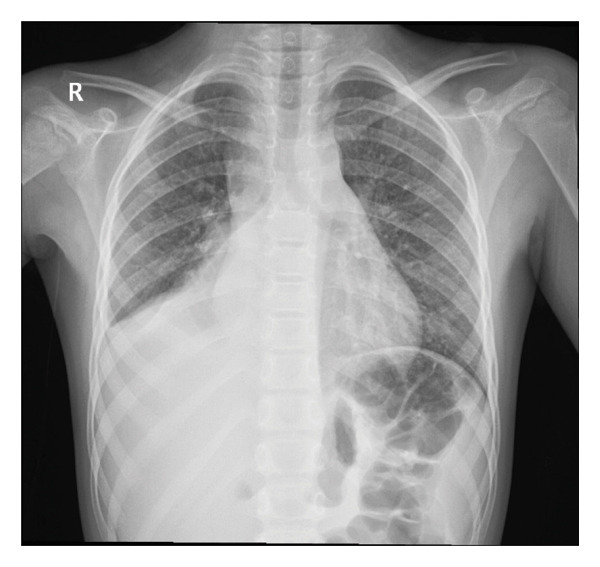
Posteroanterior (PA) chest radiograph shows increased homogeneous opacification of the right hemithorax with a lateralized pleural line and poor delineation of the right hemidiaphragm and right cardiac border, consistent with a pleural effusion.
